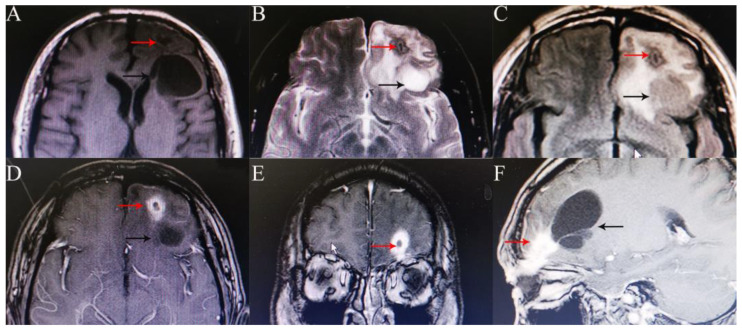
Head magnetic resonance imaging (MRI) showed a strip-shaped lesion that stretched from the left orbital roof to the left frontal lobe, was isointense on the T1-weighted image, slightly hyperintense on the T2-weighted image, and isointense/hypointense on FLARE (A–C red arrow), with obvious irregular enhancement surrounding it (D–F red arrow), and a 3.9 × 3.4 × 4.9 cm cystic shadow behind the lesion (black arrow).
